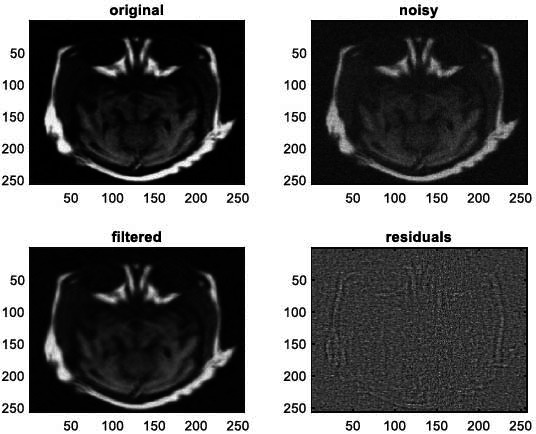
The tenth example of MRI denoising by applying the proposed image denoising technique.
